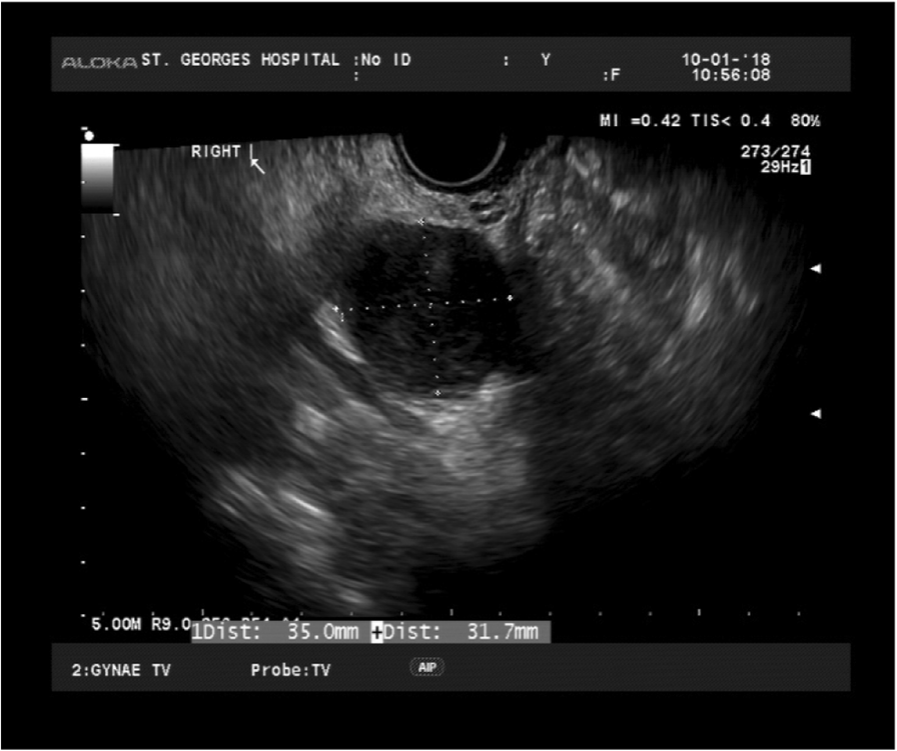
Transvaginal ultrasound image of the thrombosed right uterine artery pseudoaneuyrsm
